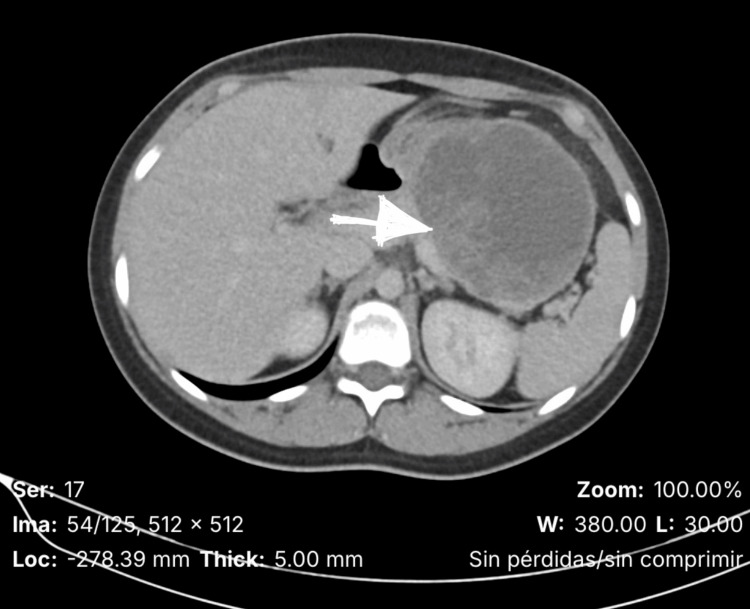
Abdominal CT Scan Showing a Well-Defined Lesion in the Pancreatic Body and Tail (Arrow Indicates the Area of Interest).
